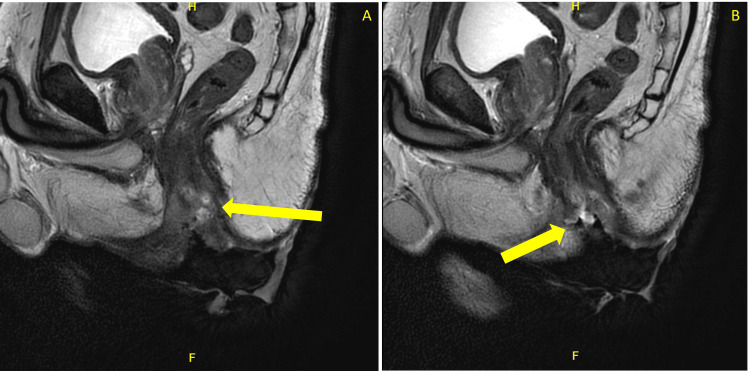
Sagittal T2-weighted pelvic MRI with contrast Sagittal T2-weighted pelvic MRI showing a heterogeneously enhancing anal canal lesion with sphincter involvement (yellow arrows). The mass distorts normal anorectal anatomy (Panel A) and extends toward surrounding soft tissues (Panel B). The letters “H” and “F” assist with image orientation and indicate head and feet, respectively.
